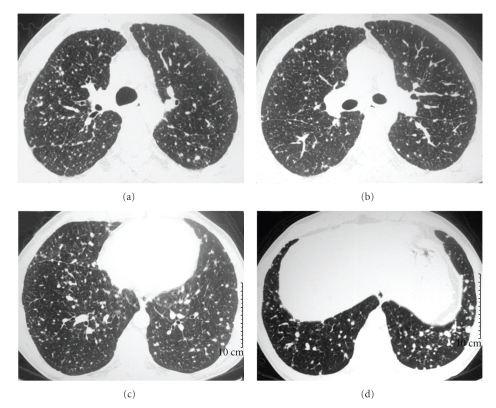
High-resolution CT scans at four different levels showing small nodules distributed randomly through the lungs. Note that the nodules are larger in the lung bases (d).
